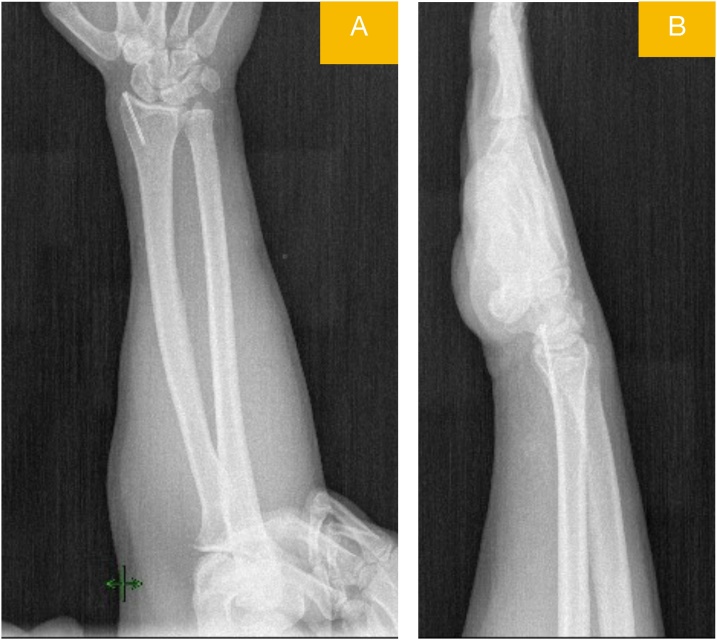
Posteroanterior (A) and lateral (B) views of postoperative second year wrist radiographs.
